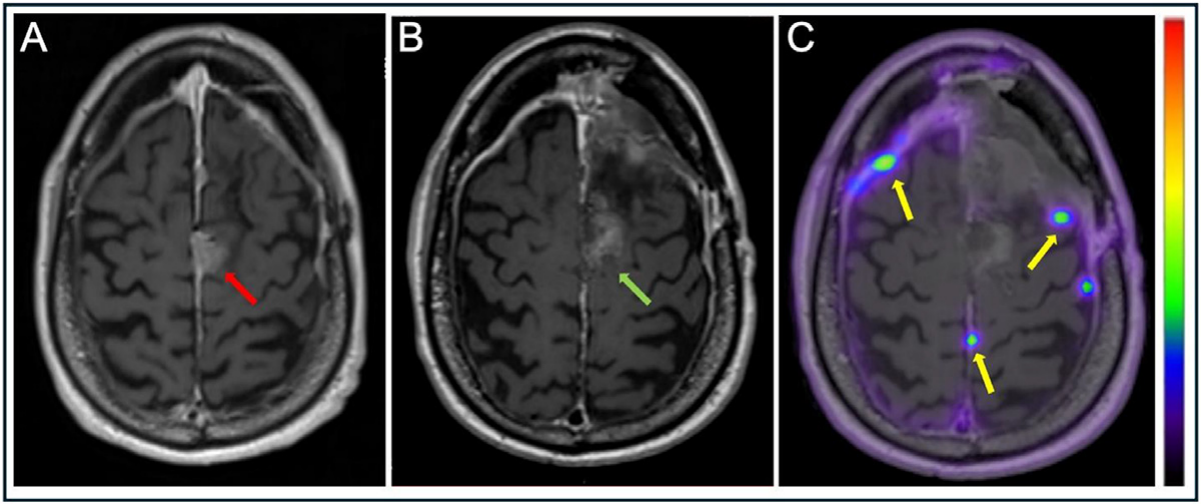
Axial T1 with contrast prior to second craniotomy (A). Overlying the left and right frontal convexity, there is an extra-axial, avidly enhancing, dural based lesion in the prior resection cavity (red arrow). The dural-based lesion caused mass effect on the subjacent brain parenchyma. Following MRI, the patient underwent resection of the recurrent dural-based mass as seen on postoperative axial T1 with contrast image (B). A focal area of enhancement in the resection cavity was suspicious for residual tumor or postoperative changes (green arrow). Pathology revelead a WHO grade III meningioma, suggesting progression of the meningioma. Postoperative axial T1 weighted MRI with contrast fused with DOTATATE PET (C, windowed SUV 0-15) demonstrates multiple residual, lesions along the dura (yellow arrows) that were not previously visualized on prior MRI with contrast.
